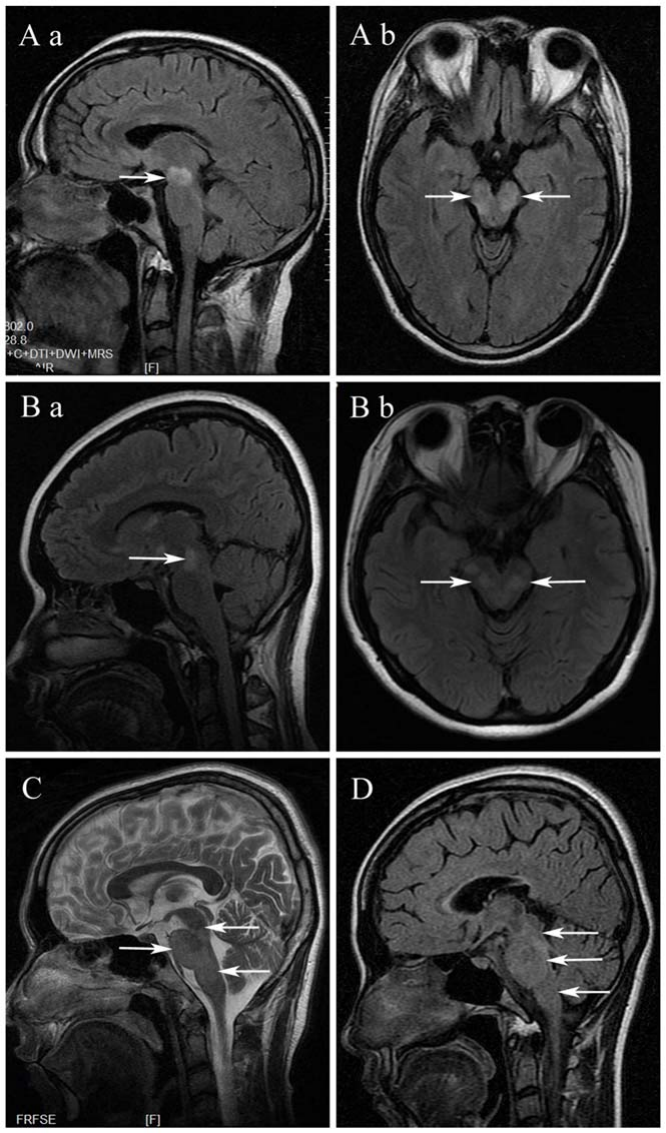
Brain stem lesions (arrows) on MRI of acute disseminated encephalomyelitis (ADEM). (A, B) Fluid-attenuated inversion recovery (FLAIR) image showing midbrain lesions with poorly defined margins located in the ventral part (symmetrical or bilateral). (C, D) Sagittal image highlighting multiple brain stem lesions (midbrain, pons, medulla) with poorly defined margins.
